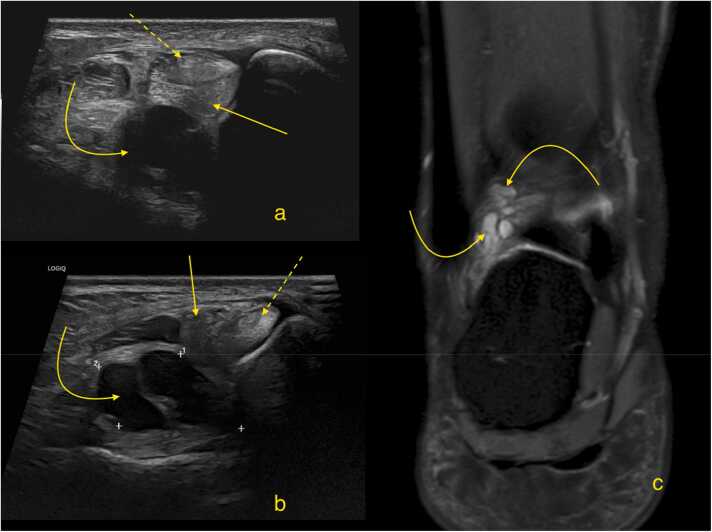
A patient with chronic pain behind the lateral malleolus. Ultrasound (a and b), transverse sections. Magnetic resonance imaging, coronal section (proton density-weighted with fat suppression, c). The peroneus brevis (straight arrow) and peroneus longus (straight dashed arrow) tendons show discrete signs of tendinopathy. A ganglion (curved arrow) is visible posterior to the peroneal tendons.
